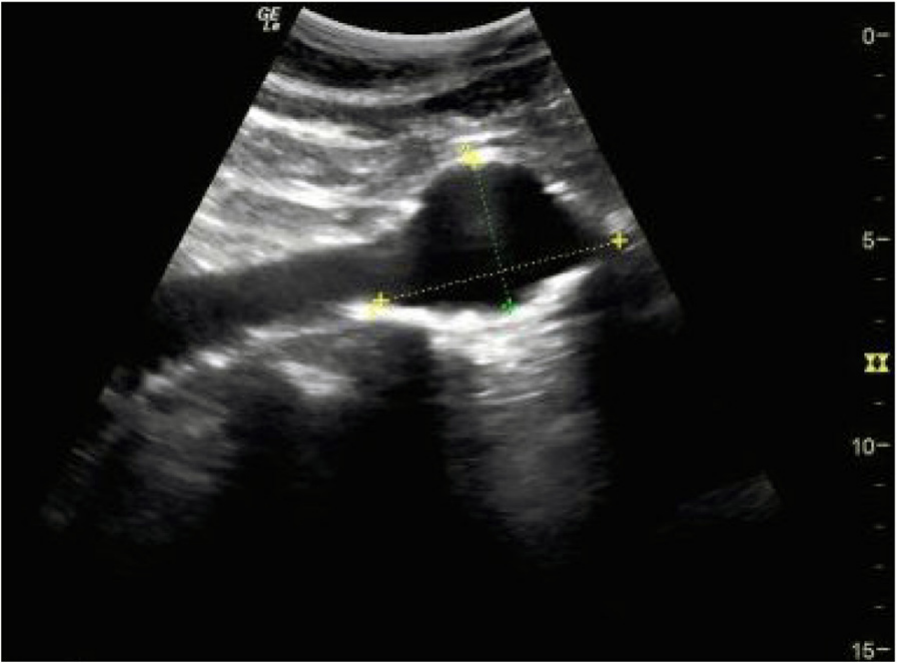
Sonography image showing an AAA in a longitudinal section. It shows the continuity of the aneurysm with the rest of the abdominal aorta
